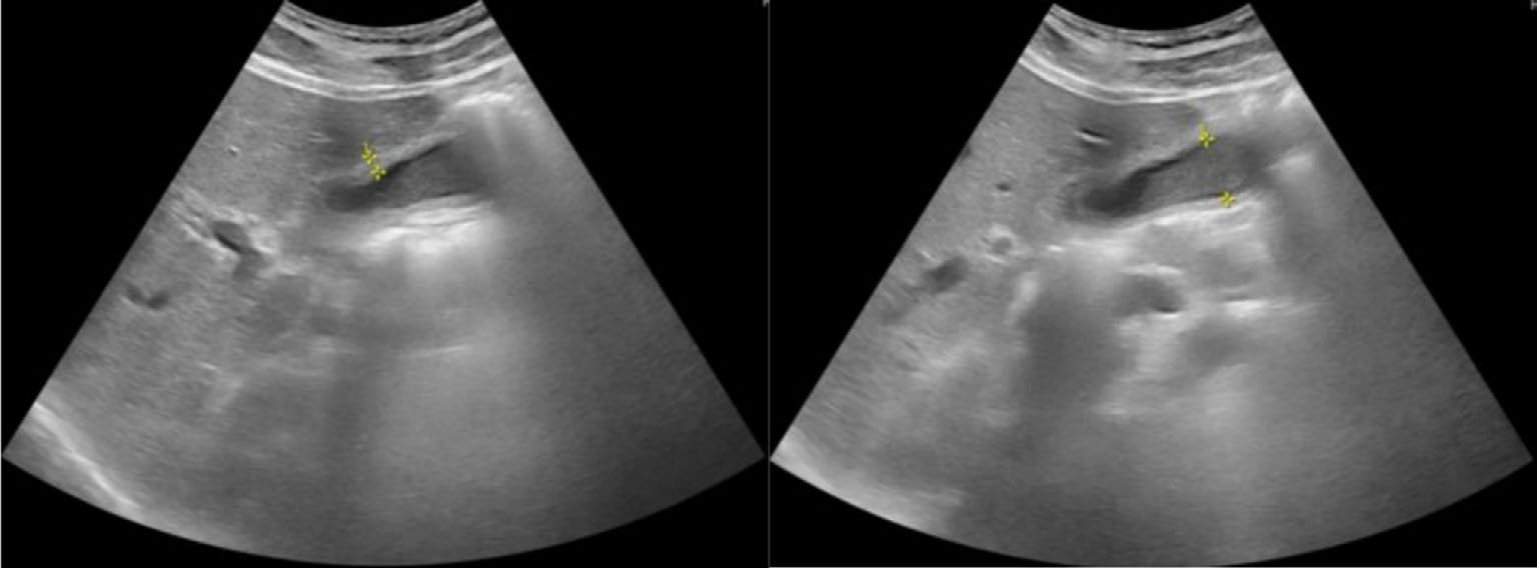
Abdominal ultrasonography demonstrates a gallbladder with significant wall thickening (measuring 15 mm) and the presence of biliary sludge. No gallstones are visualized, and there is no evidence of gallbladder distension.
